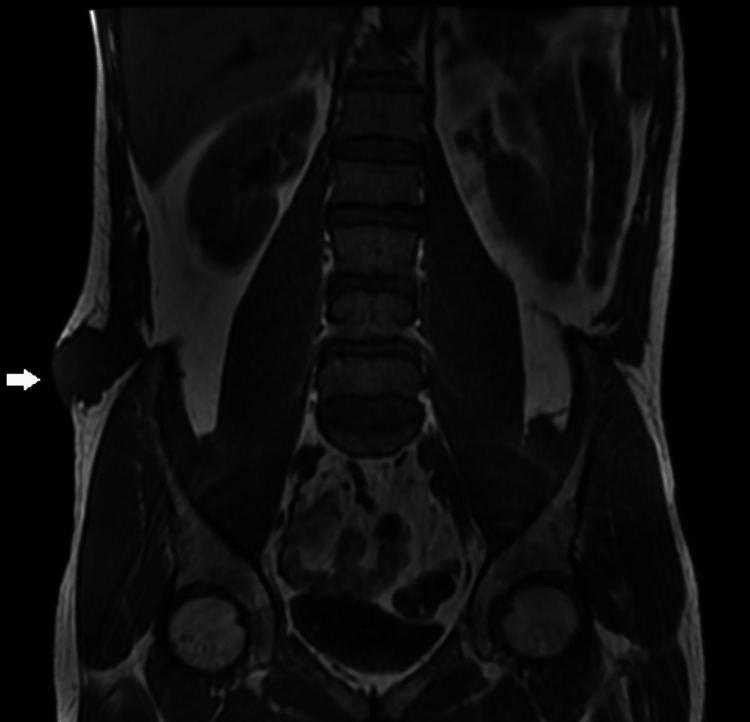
MRI image of a frontal section of the pelvis showing the tumor
